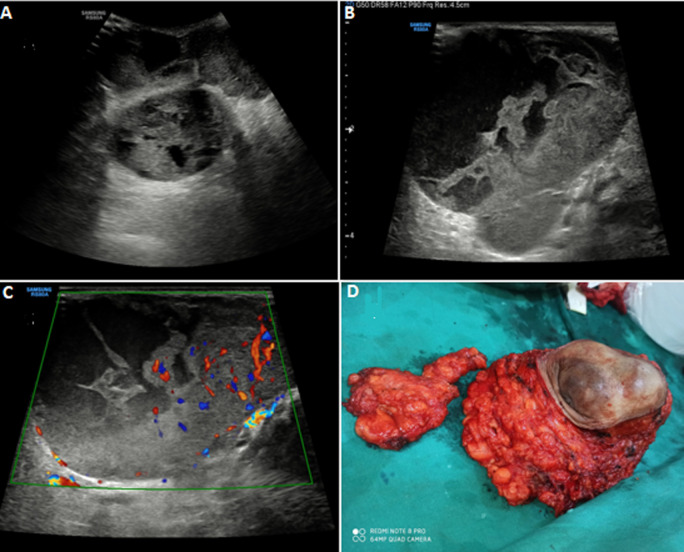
A) B-mode image using a low-frequency convex probe showing a heterogenous solid cystic lesion in left breast parenchyma; B) B-mode image using a high-frequency linear probe showing multiple echoes and septae within the cystic component; C) color Doppler image showing significant vascularity within the solid component; D) post-operative image of the resected mass
